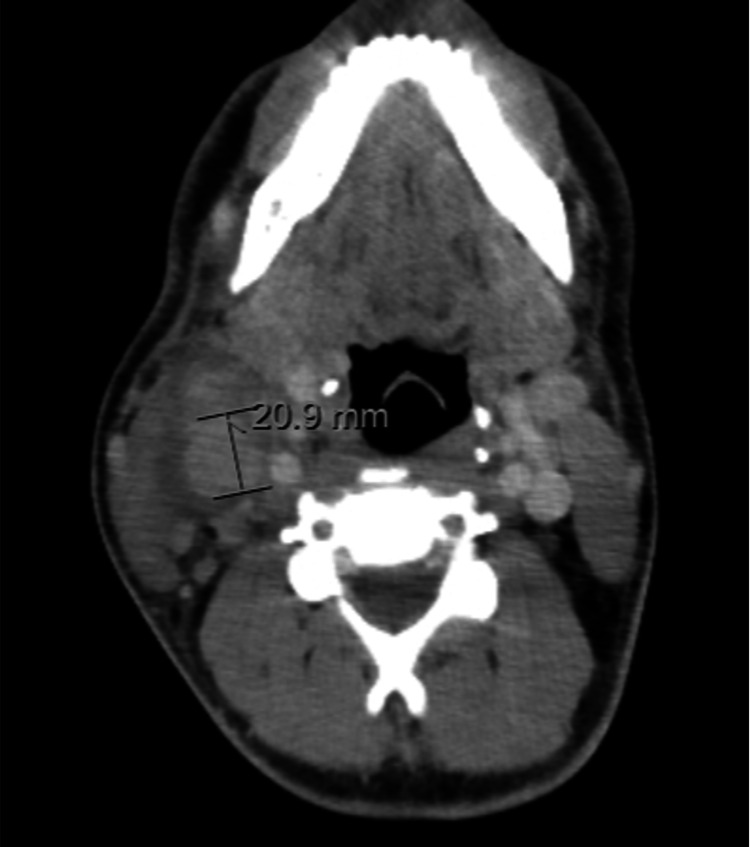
Neck CT scan Cervical adenopathy, mainly globose and atypical right jugular-carotid lymph nodes, the largest one with 21 millimeters.
